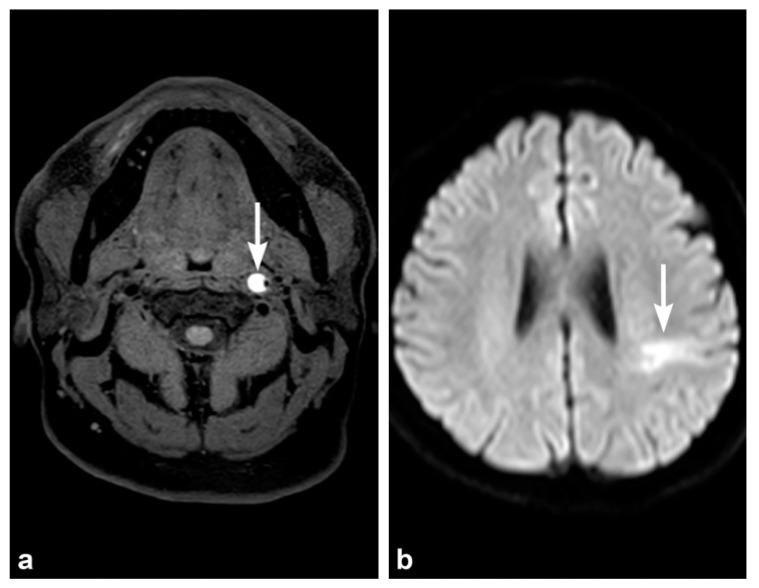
49-year-old male with left internal carotid injury and ischemic stroke (a) Axial T1-weighted magnetic resonance (MR) image shows crescentic T1 hyperintensity in the proximal left internal carotid artery compatible with acute intramural hematoma and dissection. There is increased external diameter of the left ICA with severe eccentric luminal narrowing. (b) Diffusion-weighted magnetic resonance (MR) image obtained the two days afterwards shows left middle cerebral artery territory stroke. The patient had developed right-sided weakness.
